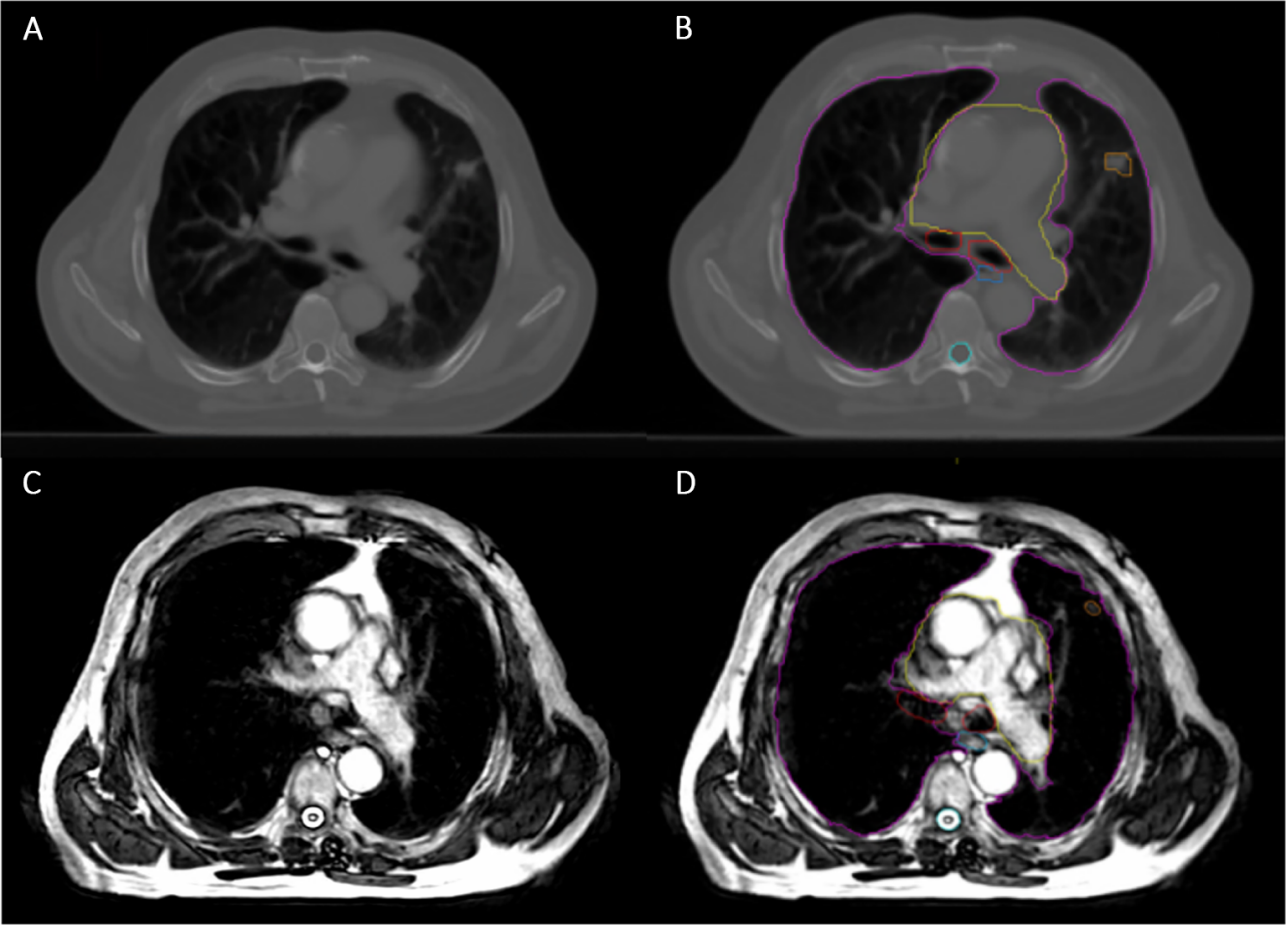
Planning Computed Tomography (CT) image compared with MR image on the Unity. (A) Planning CT image showing small peripheral right lung tumor. (B) The same planning CT image including tumor and OAR contours (pink = lungs, yellow = heart, red = proximal bronchial tree, blue = oesophagus, cyan = spinal cord and orange = Gross Tumor Volume). (C) Unity MR image for the same patient, using 3D Vane – balanced Turbo Field Echo (bTFE) sequence. (D) The same Unity MR image including tumor and OAR contours, as described before.
